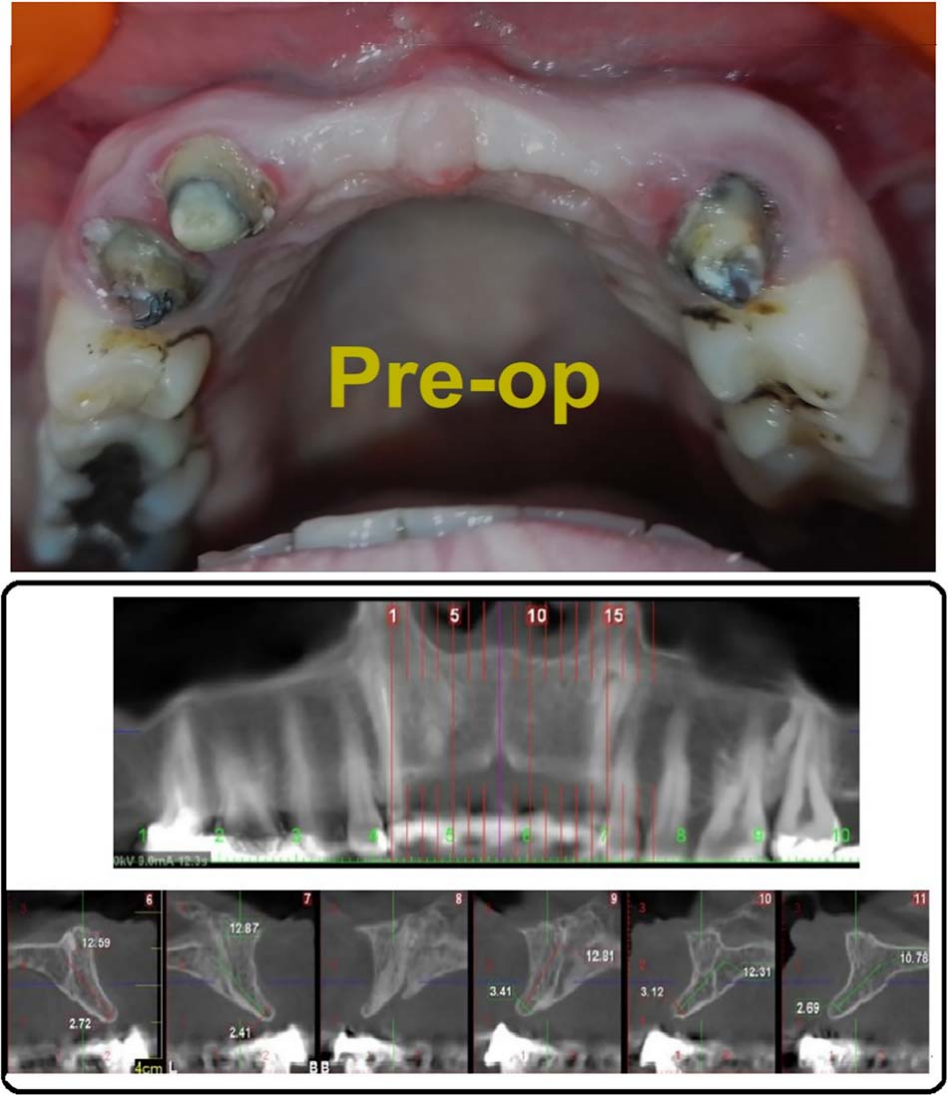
(A) Pre-operative photograph, (B) pre-operative CBCT.
